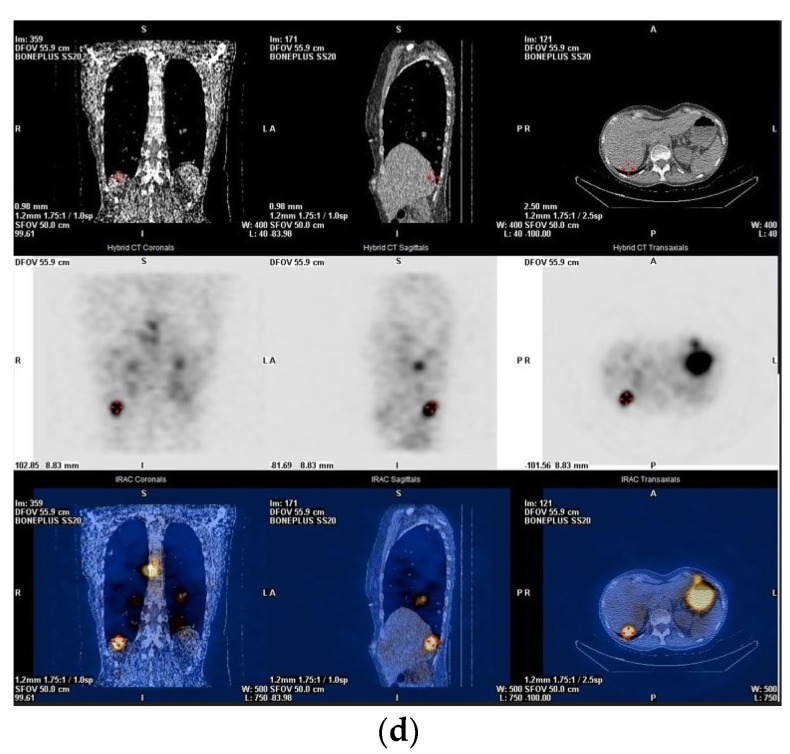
D.B., a 60-year-old woman, after total thyroidectomy and neck dissection, papillary thyroid carcinoma, initially staged pT3bN1M1; previously underwent first radioiodine treatment with 5.55 GBq I-131, then received irradiation of metastatic disease to the right neck and spine VTh5-7, and an additional course of radioiodine therapy of 7.4 GBq (200 mCi) I-131. (a) Planar PT-WBI after administration of I-131 of 7.4 GBq (200 mCi) anterior and posterior images, and post-treatment SPECT/CT images. (b) Axial CT, SPECT, and SPECT/CT images (c) and (d) coronal, sagittal and axial planes of CT, SPECT, and SPECT/CT images demonstrate multiple foci localized in the brain, bilateral lungs, mediastinal lymph nodes, and liver corresponding to the metastatic disease.
